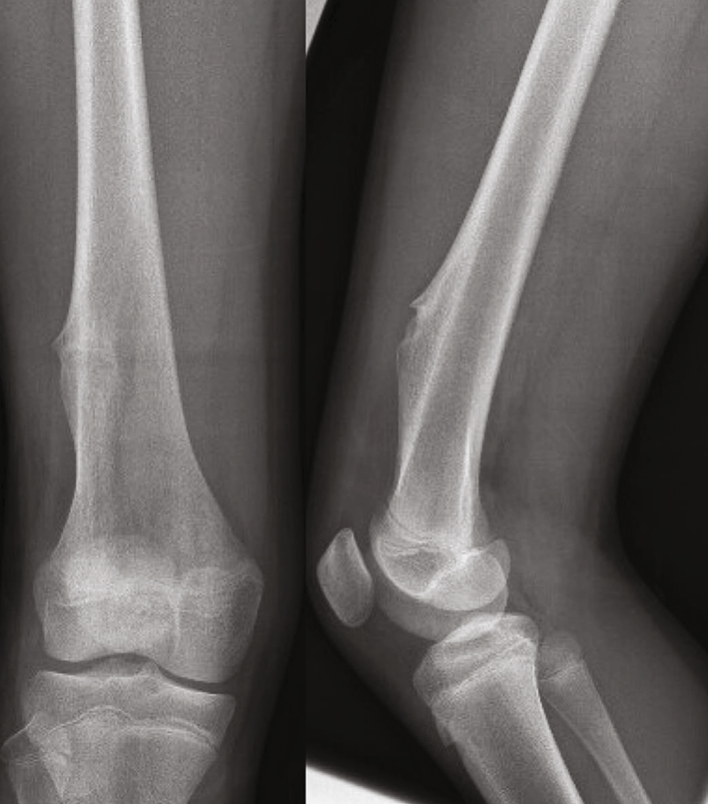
AP and lateral plain radiographs of the right distal femur demonstrate a sessile-appearing lesion on the anterolateral aspect of the distal femur consistent with osteochondroma.
